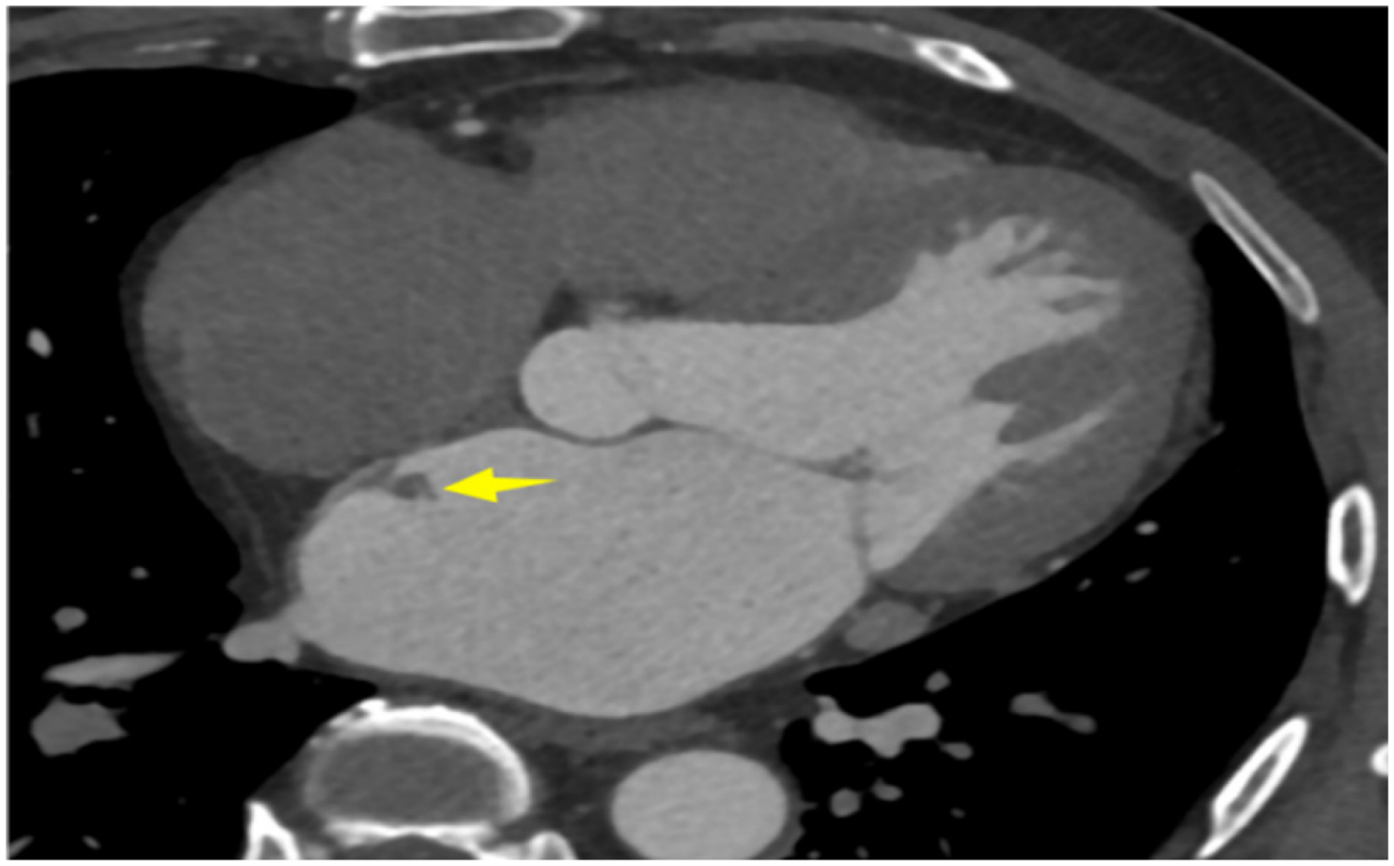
Left atrium CTA showed a mass in the left atrium arising from the atrial septal.
